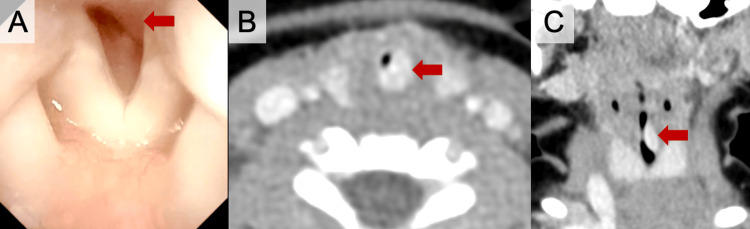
Pretreatment Laryngoscopic and CT Findings of Subglottic Hemangioma Pretreatment flexible laryngoscopy shows a smooth, compressible subglottic lesion (A), while axial (B) and coronal (C) contrast-enhanced CT demonstrate a strongly enhancing posterior subglottic mass (red arrow) causing more than 70% airway narrowing. CT, computed tomography
